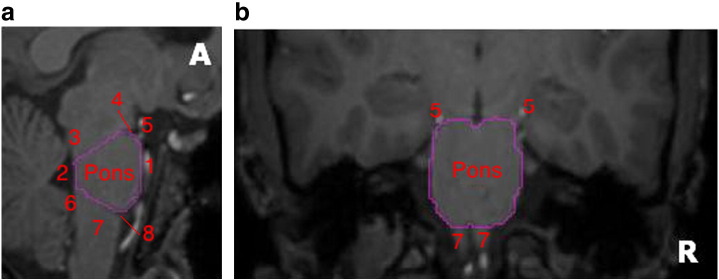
Manual delineation of the pons on MRI. (a) Sagittal view, (b) coronal view, A — Anterior, R — right. The pons was first delineated on sagittal views, followed by coronal and axial views, with the following limits; anterior/ventral: cisterna interpeduncularis and basilar artery (1); posterior/dorsal: floor of the fourth ventricle (2); superior: a line was drawn from the floor of the fourth ventricle below the superior cerebellar peduncle (3) along the lower limit of the cerebral peduncle, to the indentation between the pons and the midbrain (4); inferior: a line was drawn from the floor of the fourth ventricle above the inferior peduncle (6) to the upper limits of the olive and pyramid of the medulla oblongata (7), i.e. to the indentation between the pons and medulla oblongata (8); on coronal view: following anatomical boundaries of pons, which are clearly visible; on axial view: following delineation in both sagittal and coronal views, the pons is now clearly delineated, and the axial view is used for verification. [5 — posterior cerebral artery].
